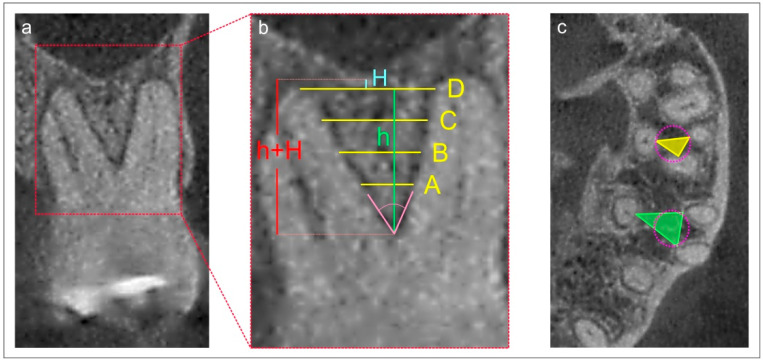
The morphometric evaluation of CBCT images of the maxillary molar region in the coronal view (CBCT image—a, with landmarks—b), and in the axial view (c). The morphometric evaluation of CBCT images in the axial view, at the C level (the triangle represents the calculated IRS surface area—green for the first molars; yellow for the second; the commonly used implant diameter in the posterior maxilla is encircled).
